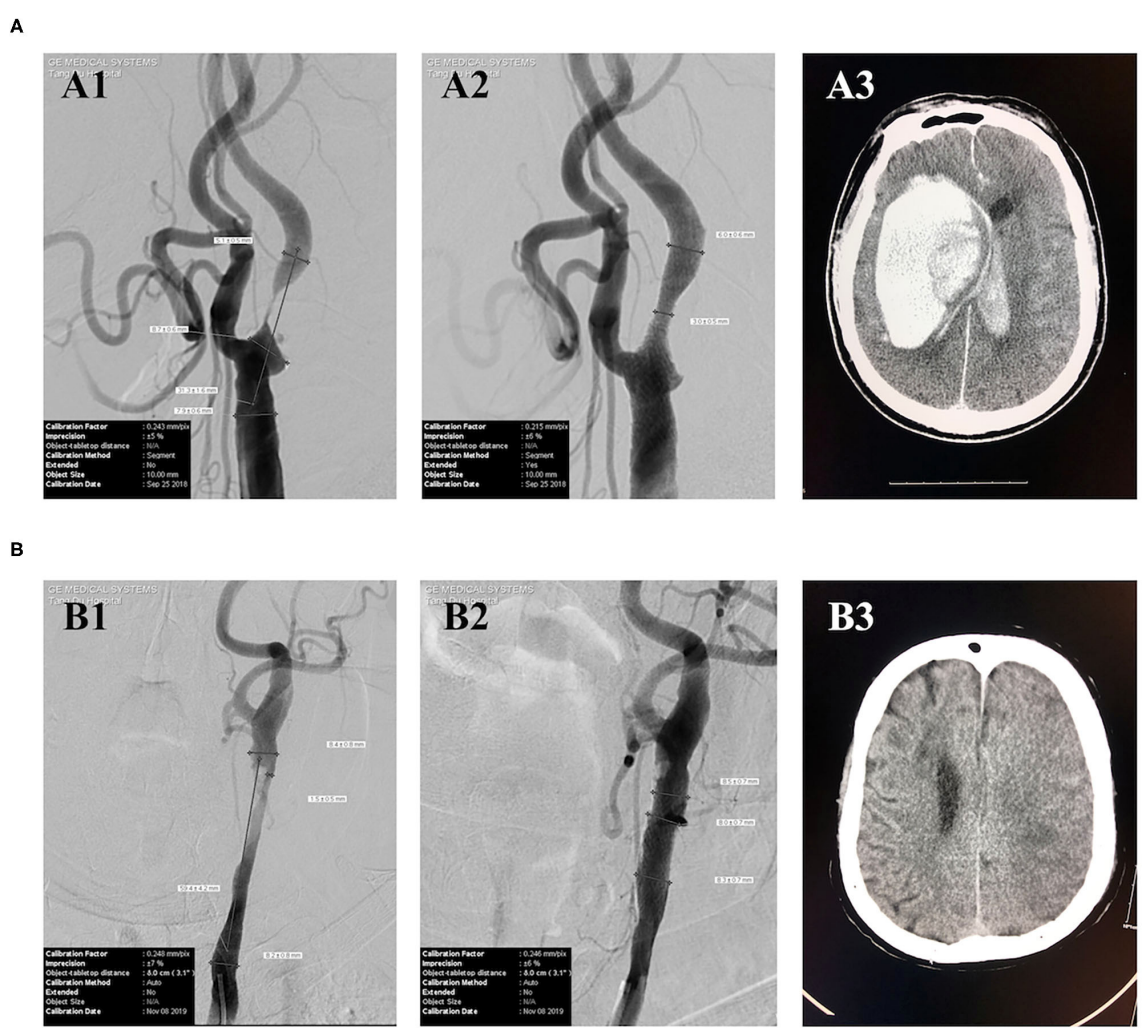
Typical cases of post-CAS ICH and CI. (A) Case #1 (A1) DSA showed a 99% stenosis in the proximal segment of the right internal carotid artery. (A2) DSA showed a 50% residual stenosis in the proximal segment of the right internal carotid artery after CAS. (A3) CT performed 25 h after CAS showed a huge right intracerebral hematoma. (B) Case #2 (B1) DSA showed an 82% stenosis in the left carotid artery. (B2) DSA showed a nearly 0% residual stenosis in the left carotid artery after CAS. (B3) CT scan performed 58 h after CAS showed a left massive CI.
